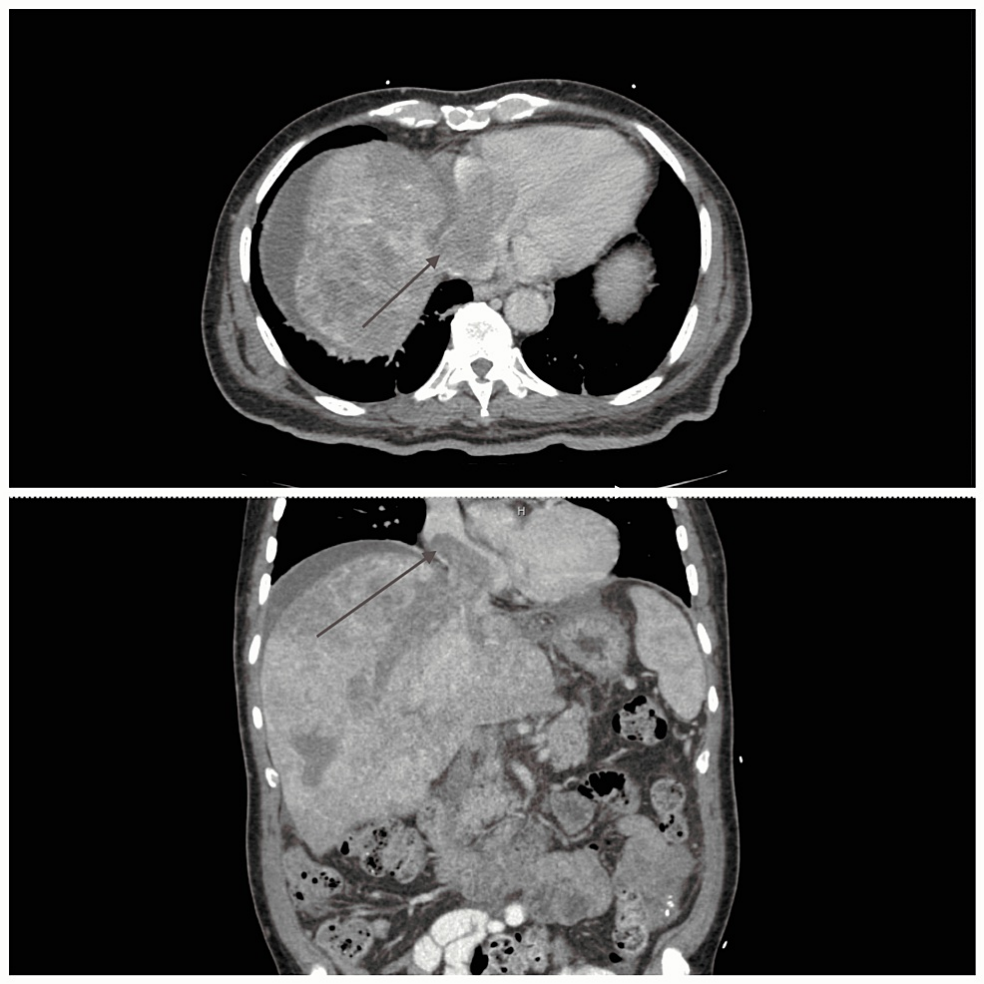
CT scan of the abdomen in the axial plane (top) and coronal plane (bottom) showing replacement of the liver with tumor. A 7cm tumor thrombus extending well into the right atrium with portal vein thrombosis (red arrow).
